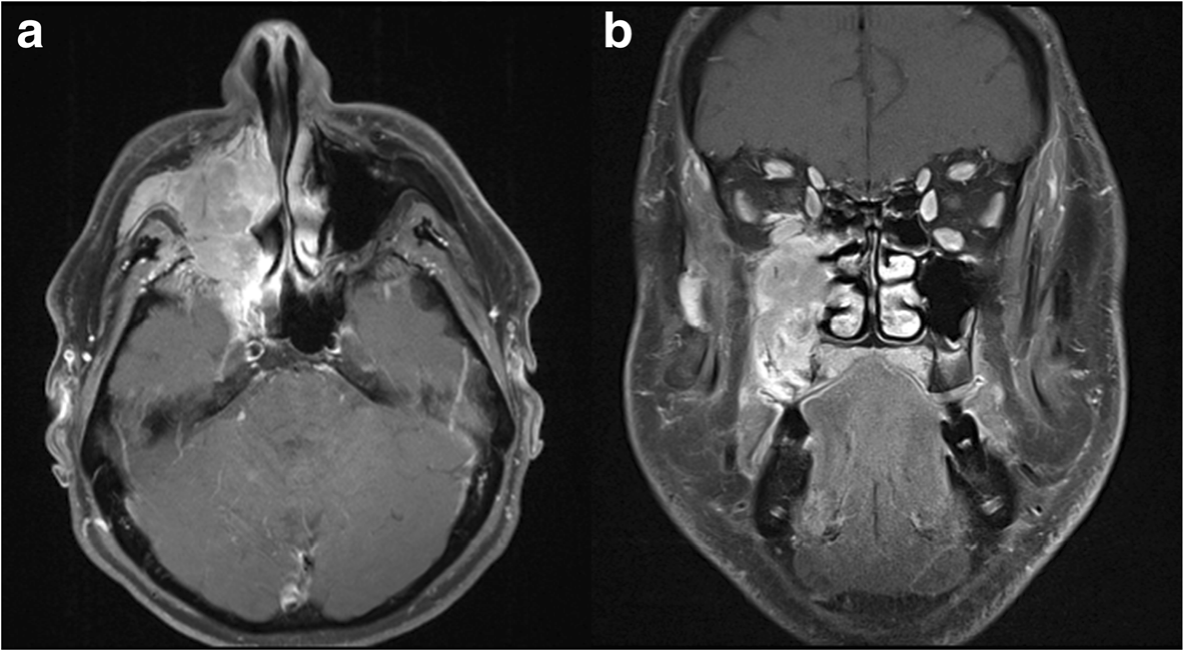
Initial, contrast-enhanced T1-weighted and fat-saturated MRI scan of the patient with mucoepidermoid carcinoma of the paranasal sinus prior to initiation of radiotherapy (a: axial; b: coronal)
